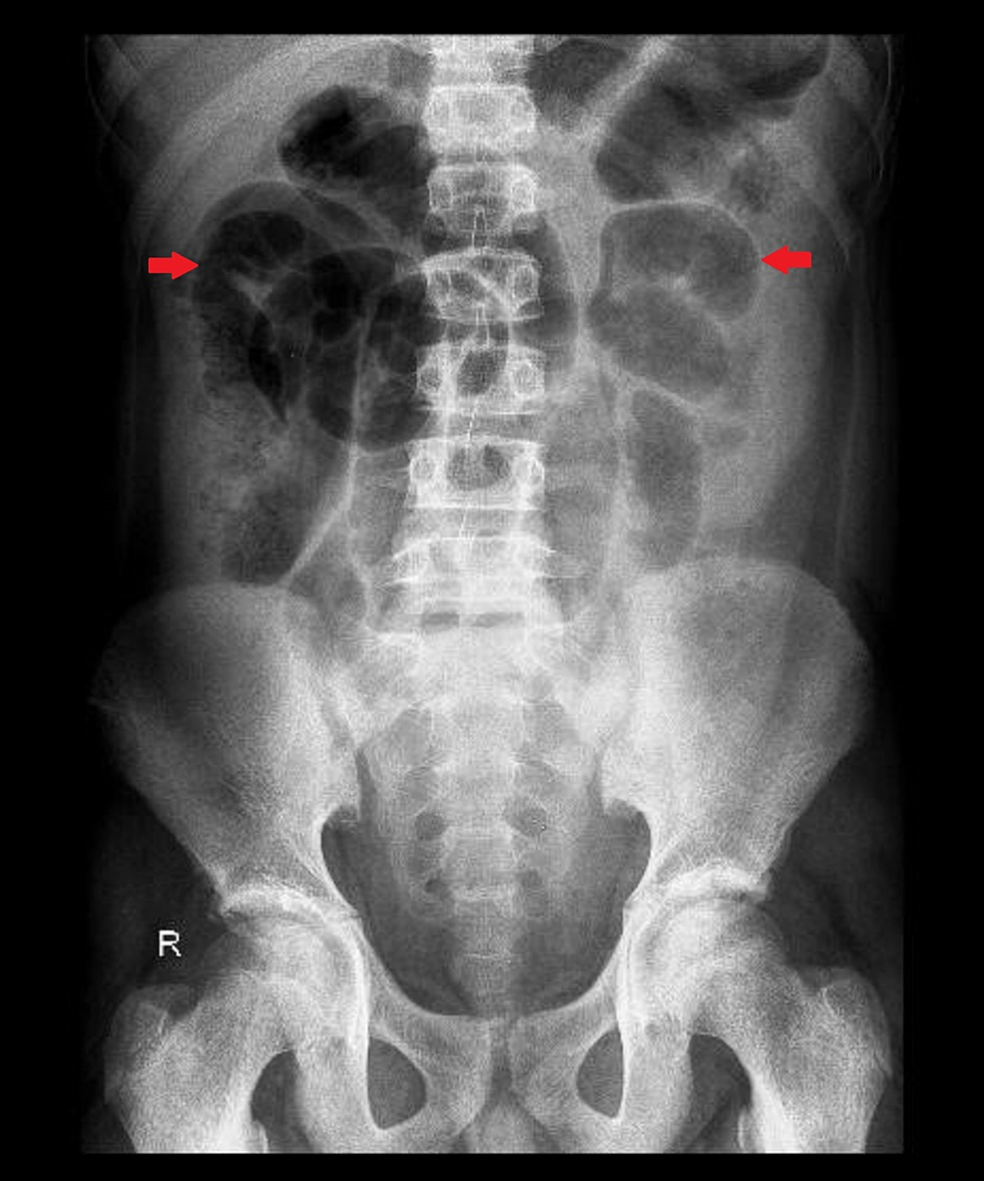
Supine abdominal X-ray showing dilated small bowel loops (arrows).
